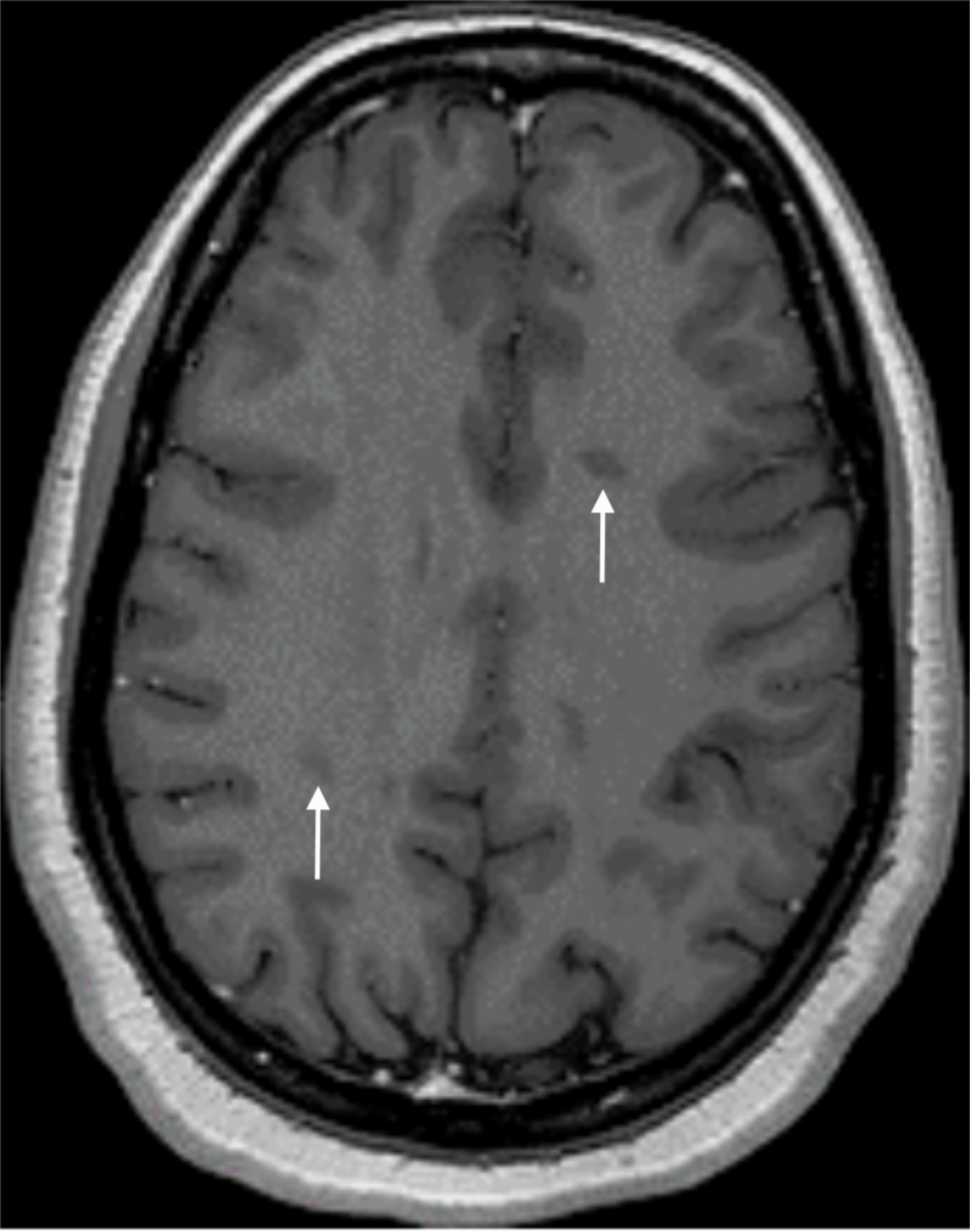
Postictal brain MRI (axial section, T1-weighted enhanced imaging) Bilateral centrum semiovale lesions, as seen in Figure 1, show no postcontrast enhancement (white arrows) MRI: magnetic resonance imaging
